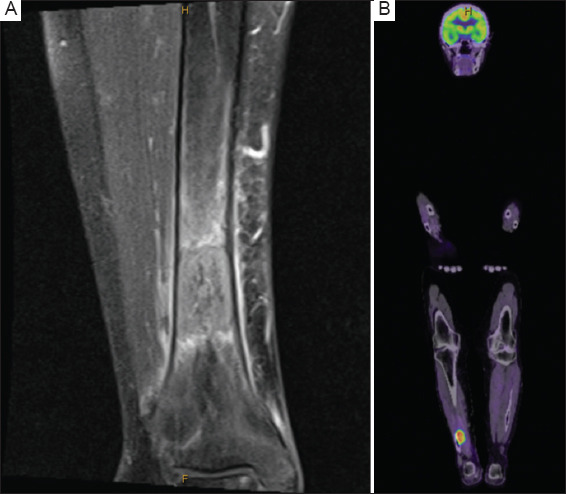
MRI and FDG PET scan of the patient. (A) An MRI scan of the right lower leg (coronal view) of the patient. The T1-weighted, contrast-enhanced, fat-saturated sequence demonstrates lesion and periosteal soft tissue enhancement. (B) An FDG PET scan (fused coronal view) of the patient Abbreviations: MRI: Magnetic resonance imaging; PET: Positron emission tomography; FDG: Fludeoxyglucose F18
